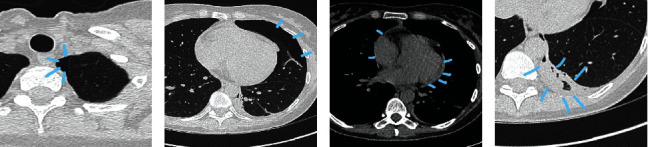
CT of patient XY chest ((a), (b) pneumothorax, (c) pericardial effusion, and (d) left lower lobe consolidation).
